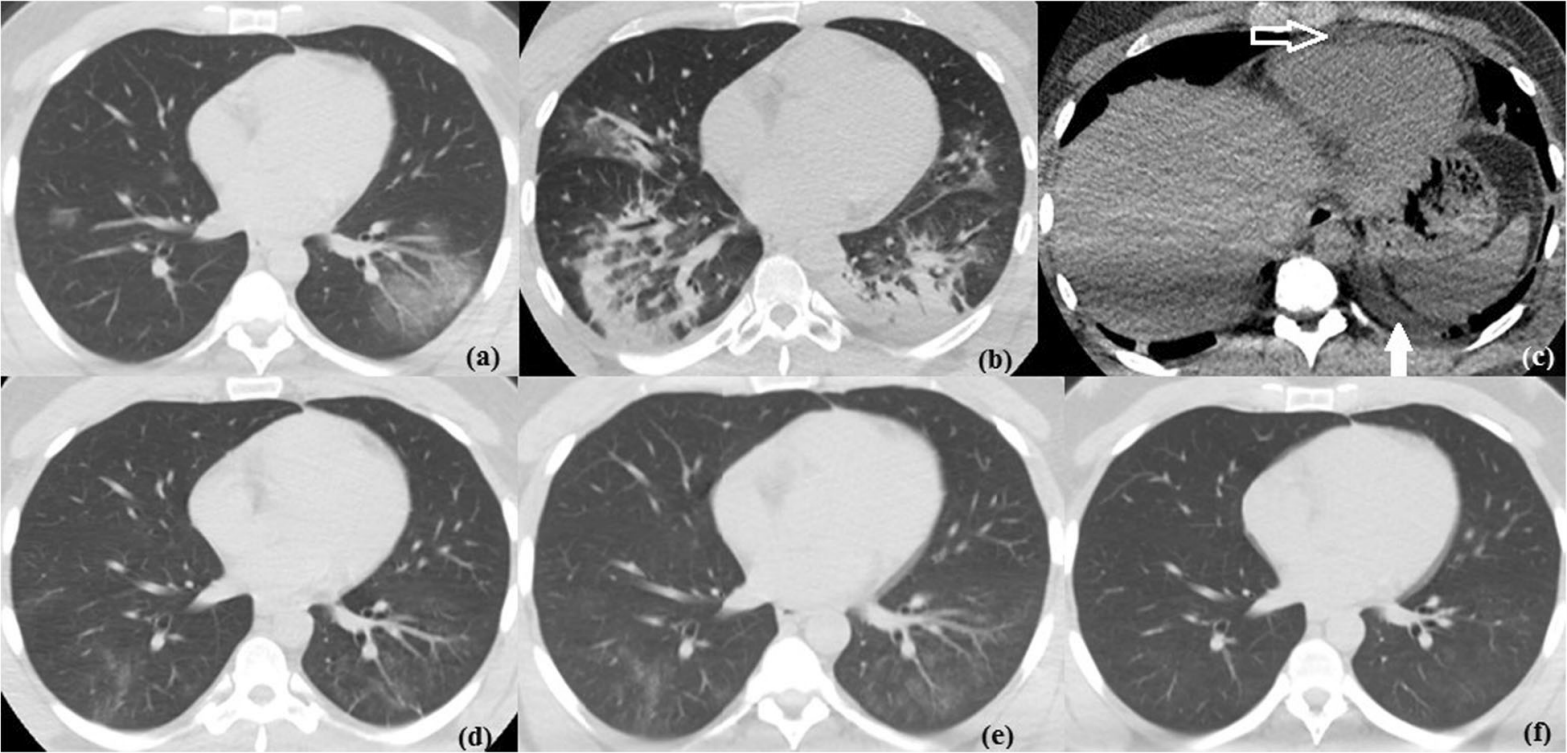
Temporal CT changes in a 35-year-old male with COVID-19. a Initial non-contrast CT chest axial lung window showing multifocal bilateral predominantly peripheral patchy ground glass opacities with vascular thickening. b, c Follow up CT study after 7 days in axial lung and axial non-contrast mediastinal windows showing progressive course regarding the extent of lung involvement with bilateral predominantly peripheral patchy consolidation and air bronchogram associated with newly developed mild pericardial effusion (hollow arrow) and mild left pleural effusion (solid arrow). d–f Follow up images 16, 22, and 28 days respectively after initial CT showing gradual regressive course with only ill-defined faint GGO seen
